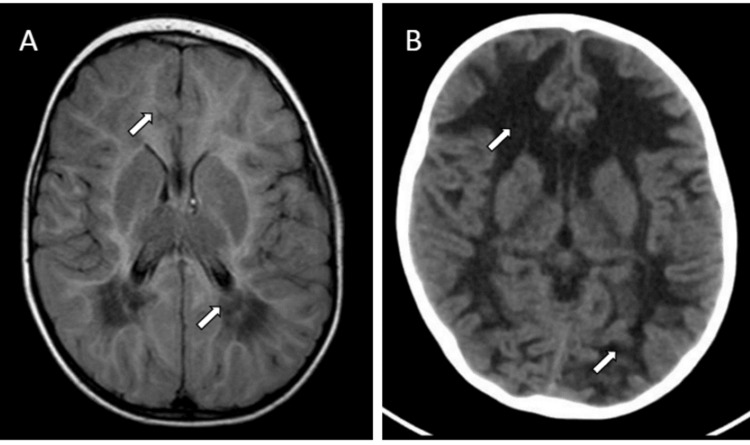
Brain imaging of patient 2 at the level of the centrum semiovale (A) Axial flair brain magnetic resonance imaging at the age of 17 months showing bilateral symmetrical diffuse confluent white matter abnormalities extending into the subcortical U fibers (upper arrow) with a high parietal tigroid appearance (lower arrow) in addition to the corpus callosum and internal capsule thinning. (B) A non-contrast axial brain computed tomography at the level of the basal ganglia, performed at the age of two years, showing further degeneration and total liquefaction of the white matter, which is more apparent in the frontal areas (upper arrow), in addition to generalized brain atrophy (lower arrow).
